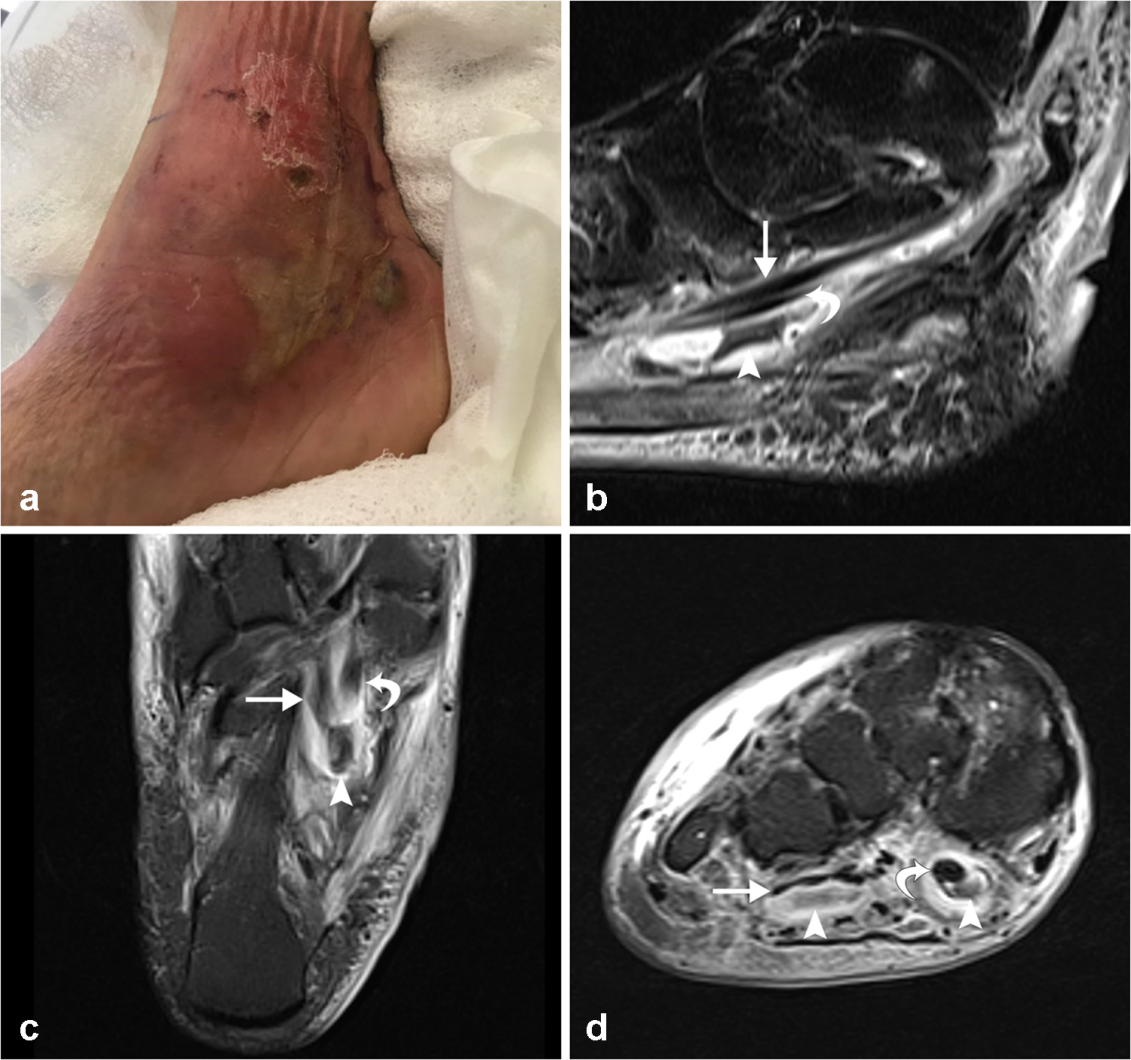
Seventy-five-year-old man with right medial ankle redness, swelling, and ulceration. Clinical photograph (a) demonstrates medial ankle cellulitis and skin breakdown. Sagittal (b), long axis (c), and short axis (d) STIR MR images demonstrate moderate to severe infectious tenosynovitis of the flexor digitorum longus (arrows) and flexor hallucis longus (curved arrows) tendons and at the Master knot of Henry with intermediate to hypointense debris (arrowheads) within the tendon sheaths
